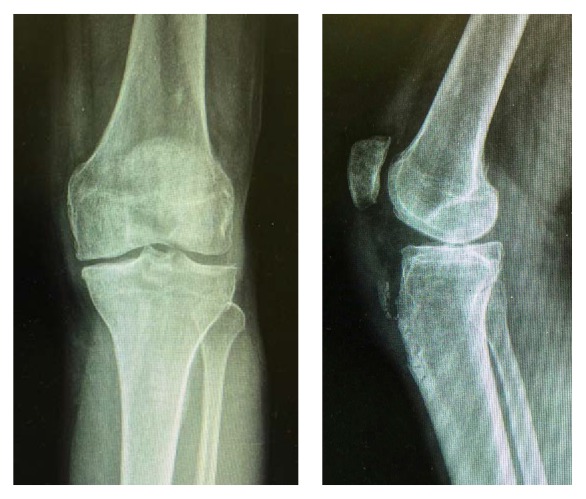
Knee X-ray at 5-month follow-up.
